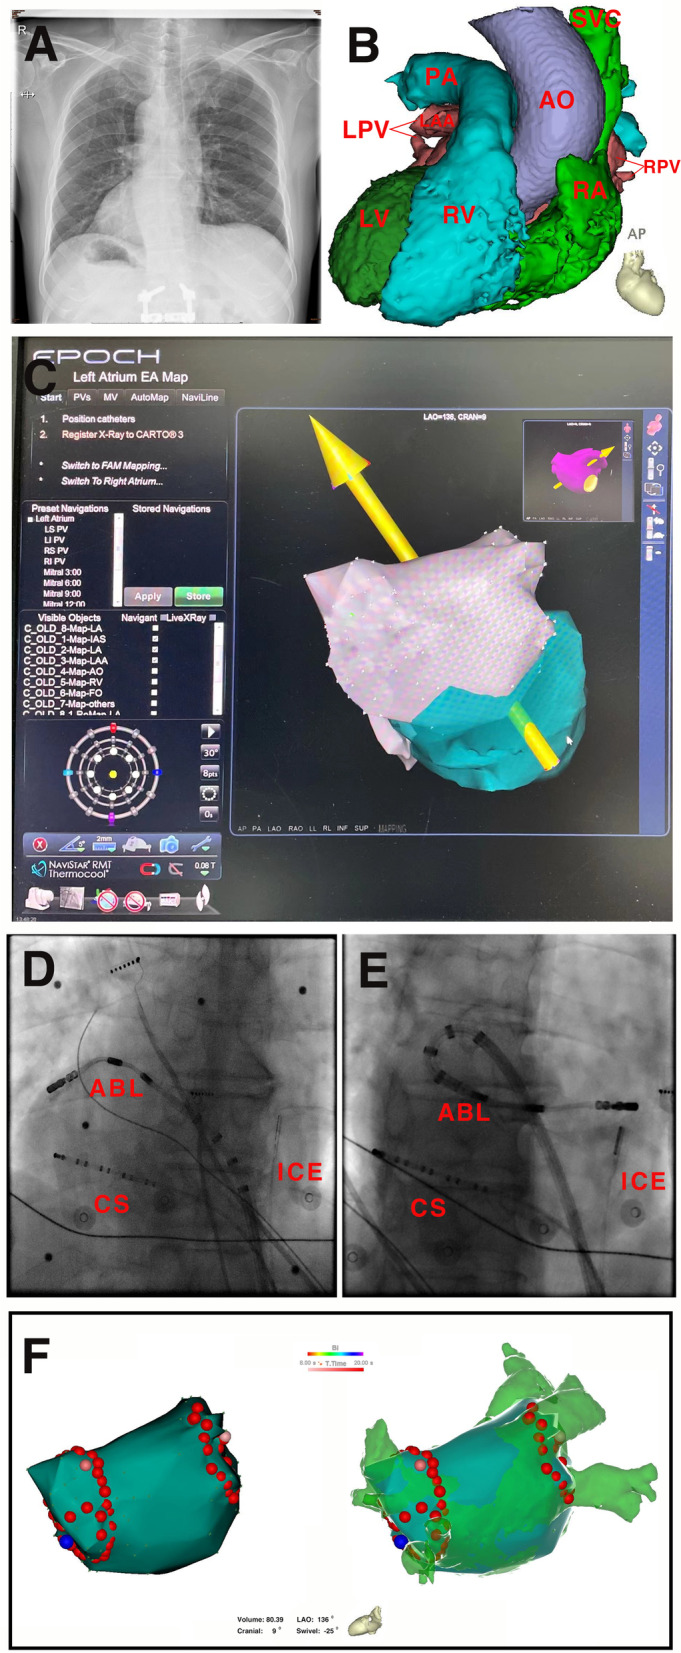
Panel A Chest radiograph reveals DSI with left-sided liver and right-sided stomach. Panel B Cardiac CT scan shows the inferior and superior vena cava connecting to the RA on the left side, and the structure and position of all four cardiac chambers presents a mirror image of a normal heart.Panel C The 3D anatomical reconstruction of the LA acquired with ICE is merging with reconstructions by the ablation catheter in the RMN system. Panel D The magnetic ablation catheter is positioned in the left inferior PV in RAO view, with a multipolar electrode catheter in CS and ICE catheter at mid-RA. Panel E The magnetic ablation catheter along with the steerable sheath forms a large loop in LA when ablating right PV in RAO view. Panel F The 3D electroanatomical reconstruction of the LA and PVs is created using the CARTO and RMN system, merging with pre-acquired CT image. Red dots indicate the ablation line around the bilateral PVs
